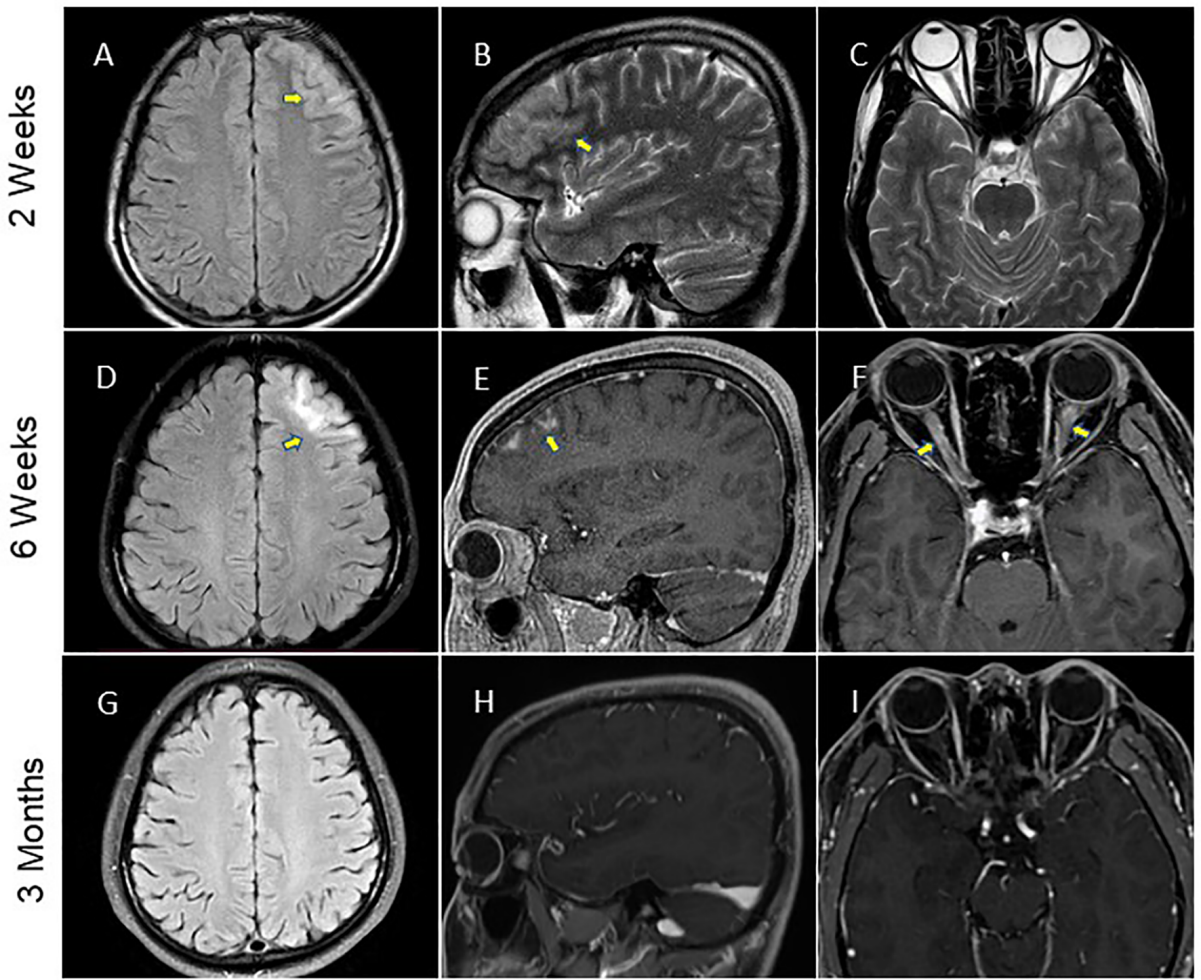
Brain MRI performance. (A–C) The MRI data of patient at two weeks after the symptom onset showed hyperintensity of the left frontal lobe on fluid-atten uated inversion recovery (FLAIR) imaging and T2-weighted imaging (arrows). (D–F) MRI of the brain was repeated at 6 weeks and showed enhancement of the left frontal lobe lesion involved the bilateral optic nerve (arrows) on FLAIR imaging conducted with gadolinium contrast. (G–I) Brain MRI scan performed at three months after initial symptom onset depicted a significant improvement of the imaging abnormality.
